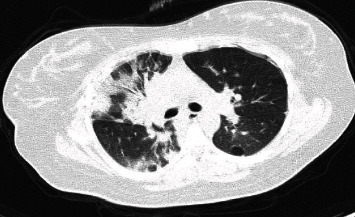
Chest computed tomography scan of the mother.
